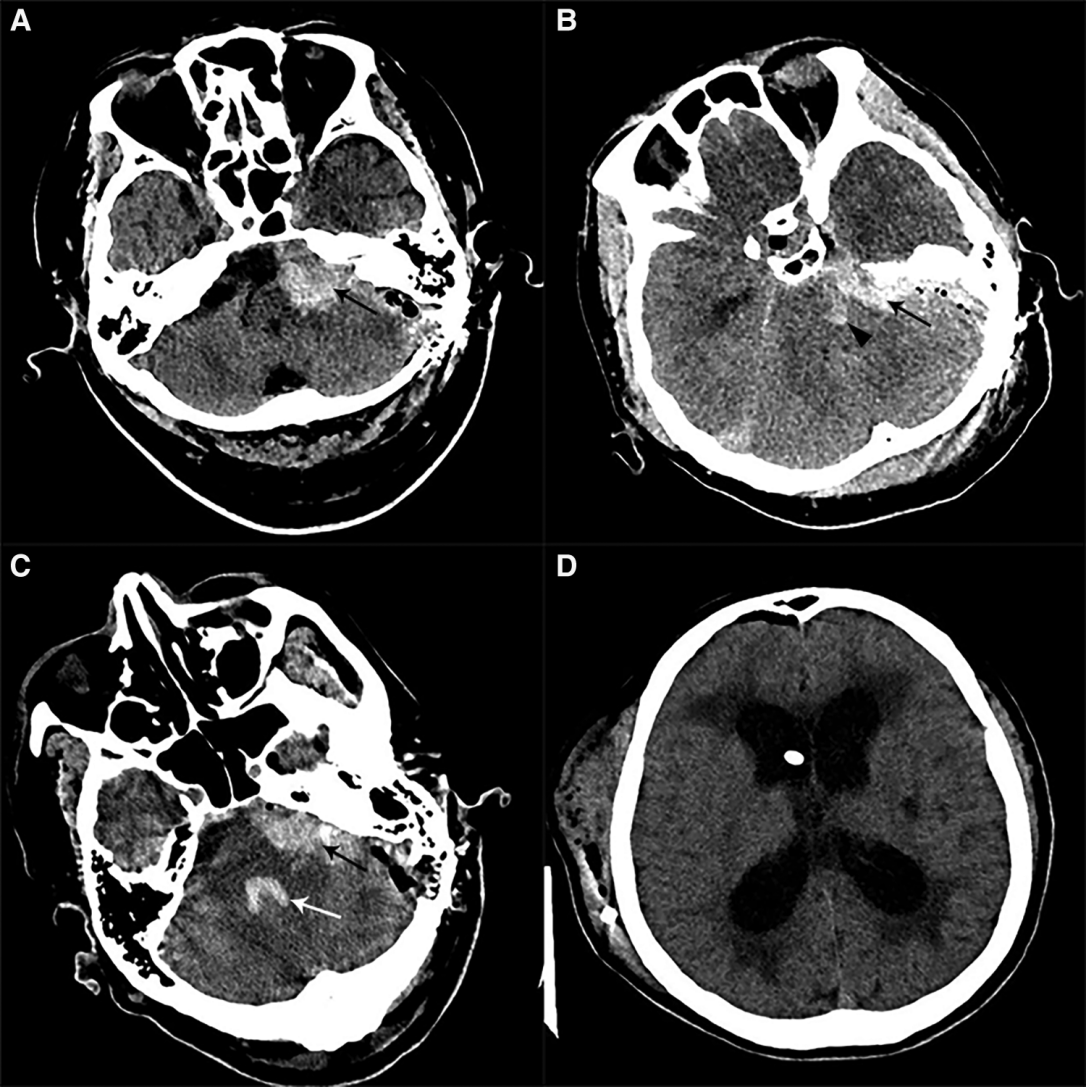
Postoperative imaging of patients with CPA hemorrhage. (A) Postoperative CT in a patient with left HFS showed a left CPA hemorrhage alone (black arrow). (B) Postoperative CT in one patient with left HFS showed CPA hemorrhage (black arrow) combined with brainstem hemorrhage (black arrowhead). (C) Postoperative CT in a patient with left HFS and TN showed a left CPA hemorrhage (black arrow) involving the fourth ventricle (white arrow). (D) We performed a V-P shunt on the patient who had experienced hydrocephalus two months after the CPA hemorrhage. CT showed a lateral ventricle enlargement, periventricular hydrocephalus, and distal stoma of the duct.
